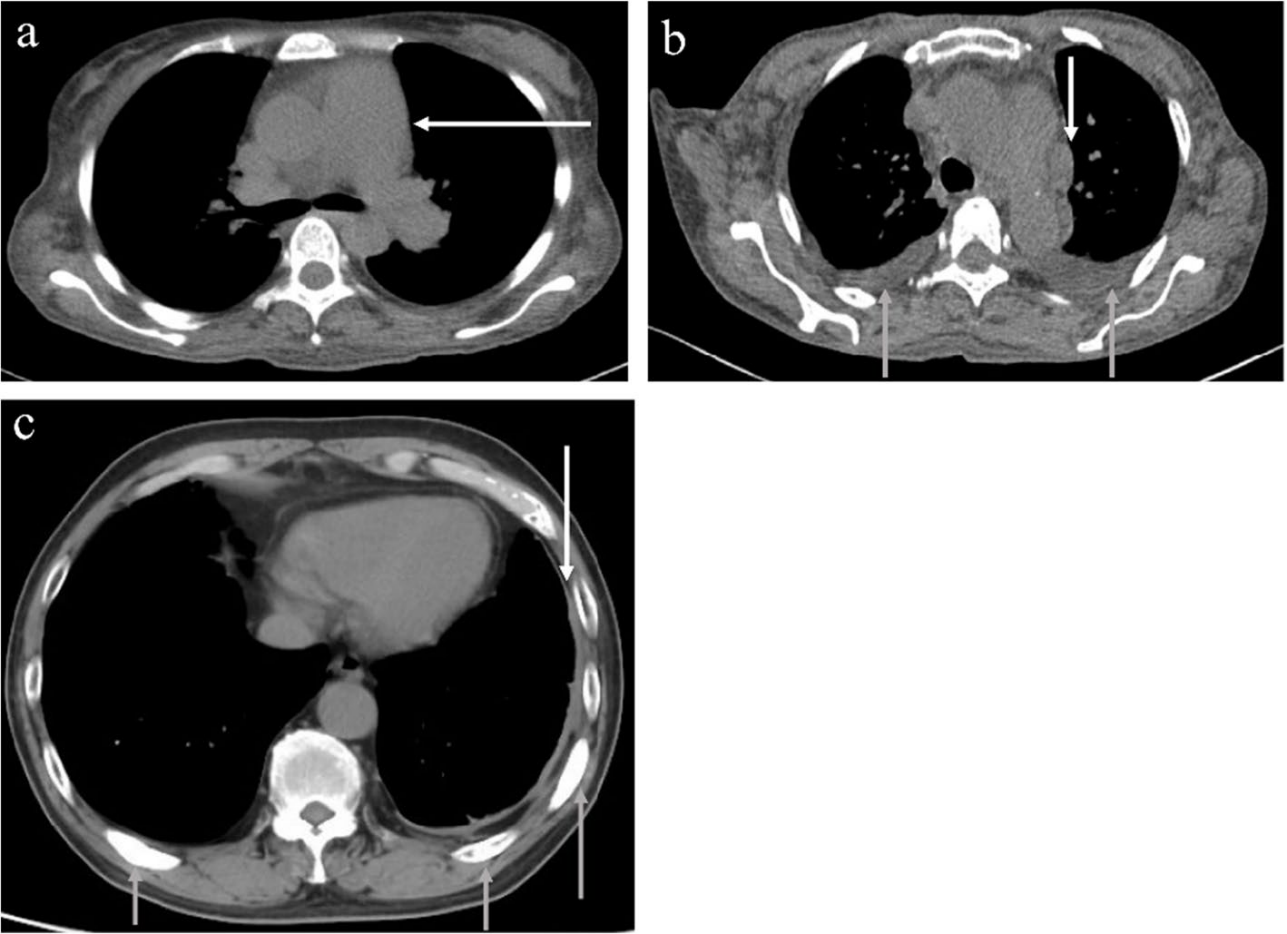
Chest CT of patients with POEMS syndrome. a A 39-year-old woman with pulmonary artery widening (white arrows). b A 53-year-old woman with an enlarged lymph node (white arrows), and bilateral pleural effusion (gray arrows). c A 67-year-old man with pleural thickening (white arrows), and bone lesions (gray arrows)
